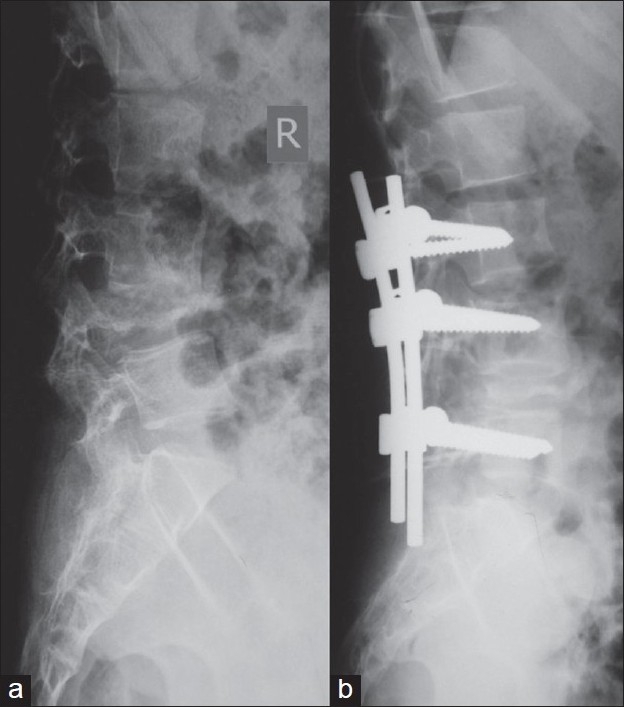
Lateral X-ray of LS spine (a) of a 15-year-old patient with TB spine lesion of L4–5 with kyphosis of 25°. The lumbar kyphosis was corrected by pedicle subtraction osteotomy and fixed with pedicle screw (b) and kyphus is corrected to 0°
